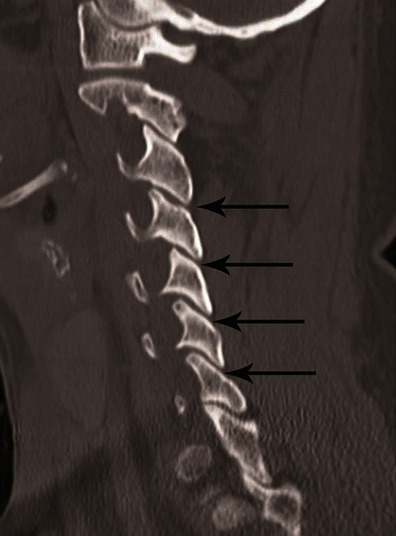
Normal alignment of facet joints on parasagittal CT image (*arrows*). A facet joint is formed by the obliquely oriented rhomboid-shaped articular processes and overlaps like a roof shingle. The inferior articular process of the cranial vertebra is posterior to the superior articular process of the caudal vertebra. On sagittal images, a facet joint articular surface should be parallel, cranio-caudally symmetrical and congruent; the inferior articular facet should cover the entire articular surface on the superior articular facet below. The facet joint gap should be less than 2 mm
